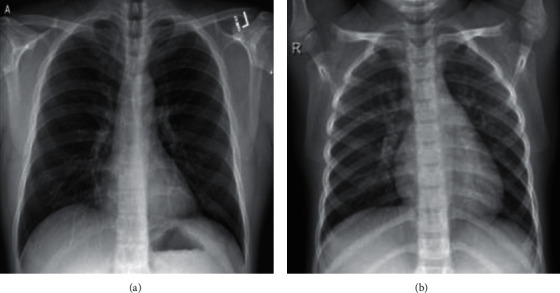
Example of X-ray image from patients' lungs. (a) COVID-19 patient. (b) Other patients.
